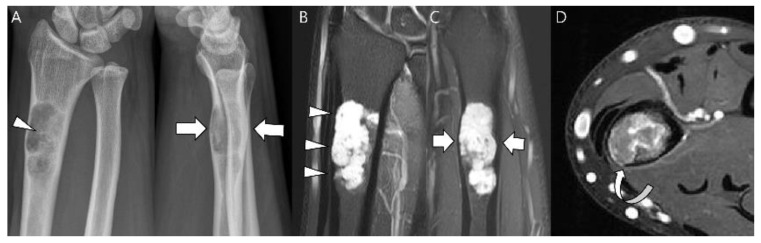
A 35-year-old man presenting with wrist pain. (A) Plain radiographs reveal a lobulated lytic lesion with chondroid matrix mineralization (arrowhead) and bone expansion (arrows) in the distal radius. (B,C) Coronal and sagittal T2-weighted fat-suppressed images show an intramedullary high signal mass with deep and extensive endosteal scalloping (arrowheads) and bone expansion (arrows). (D) Axial T1-weighted enhanced image shows peripheral rim and septal enhancement. Note the volar cortical thinning or defect (curved arrow). This lesion was noted as an atypical cartilaginous tumor at the initial incisional biopsy but was revealed as chondrosarcoma grade 2 at extended curettage.
